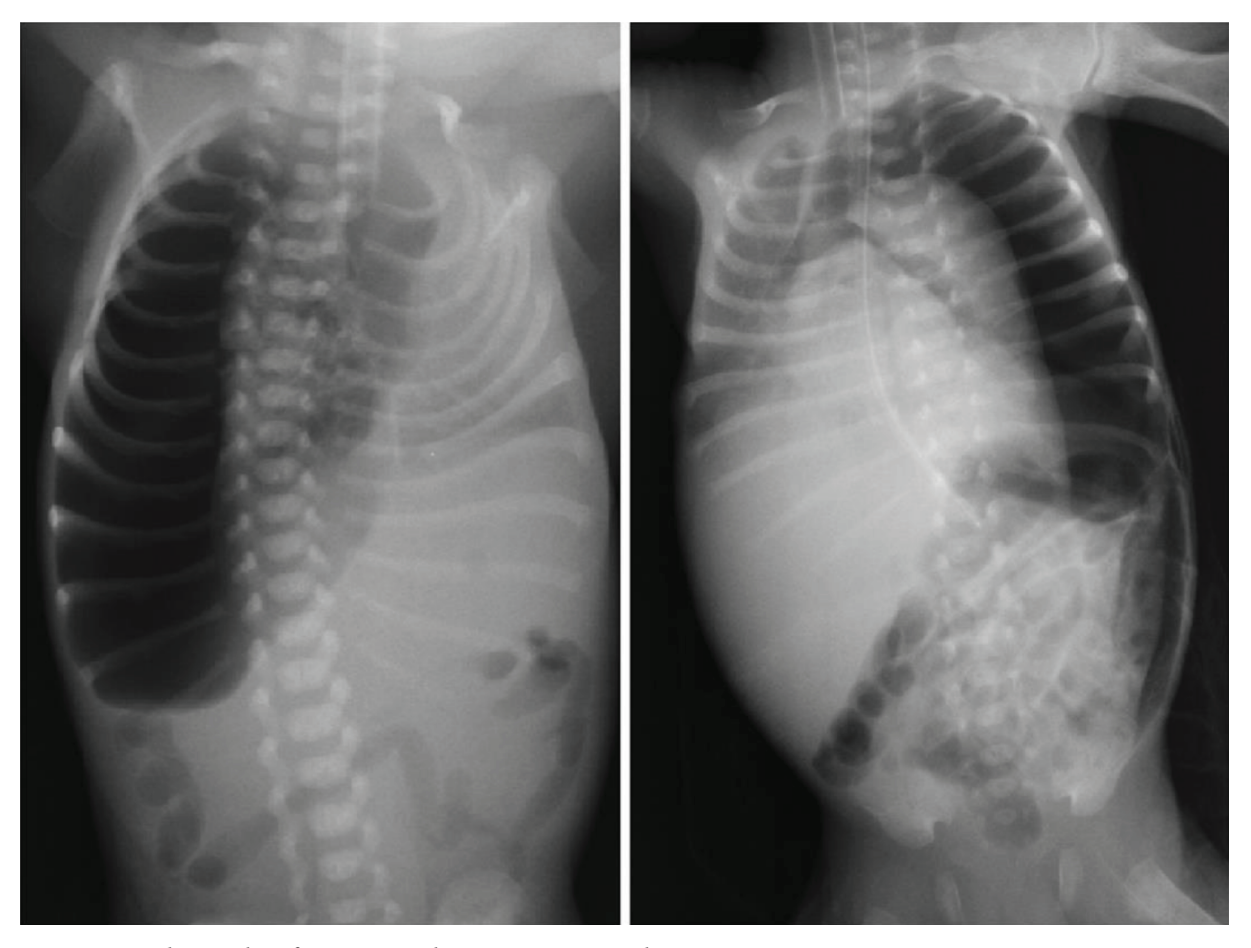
Radiographs of patients with type III pneumothorax.
